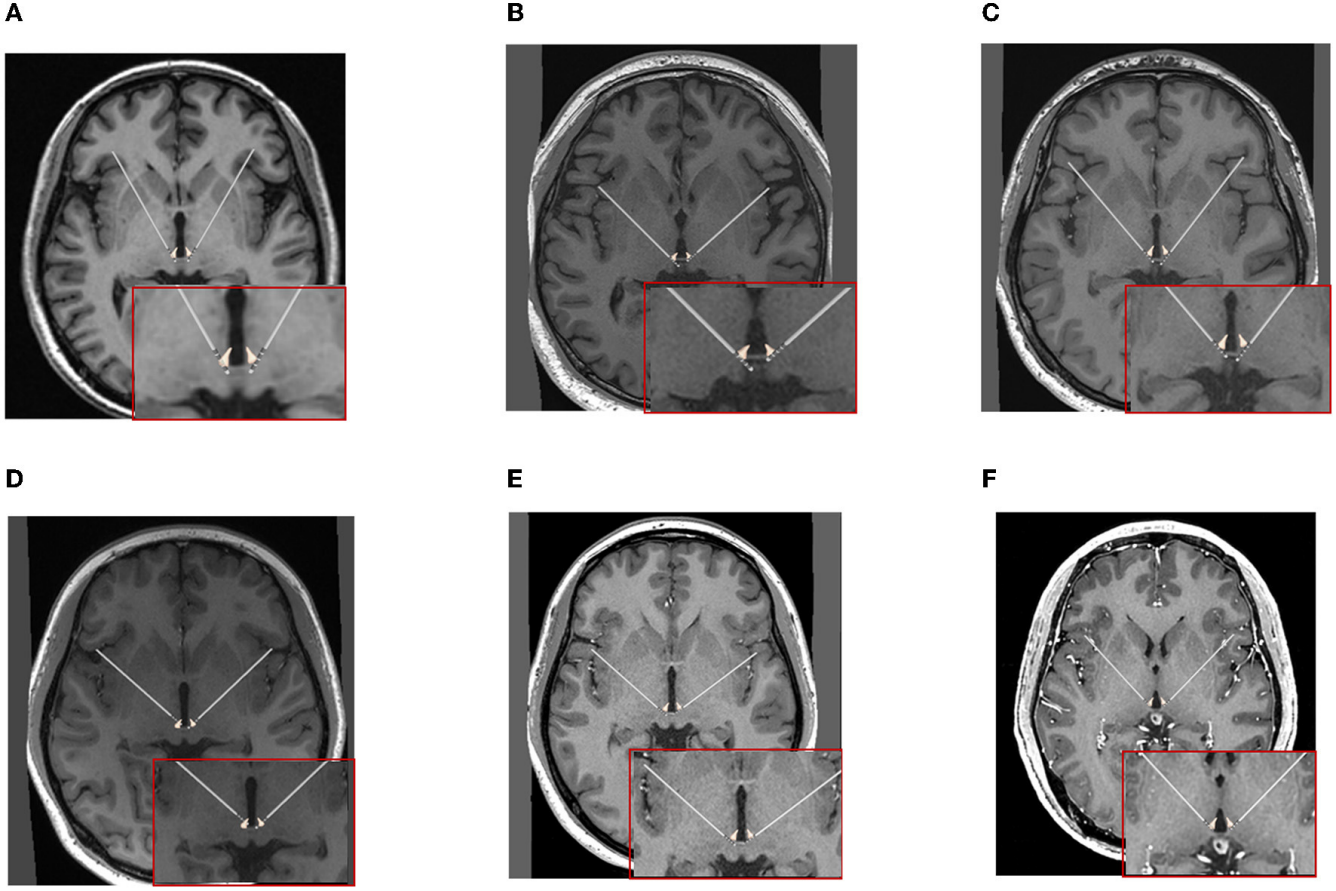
The positions of DBS electrodes were reconstructed with the preoperative MRI and CT at 1 month after surgery for each patient. (A–F) Cases 1–6.
